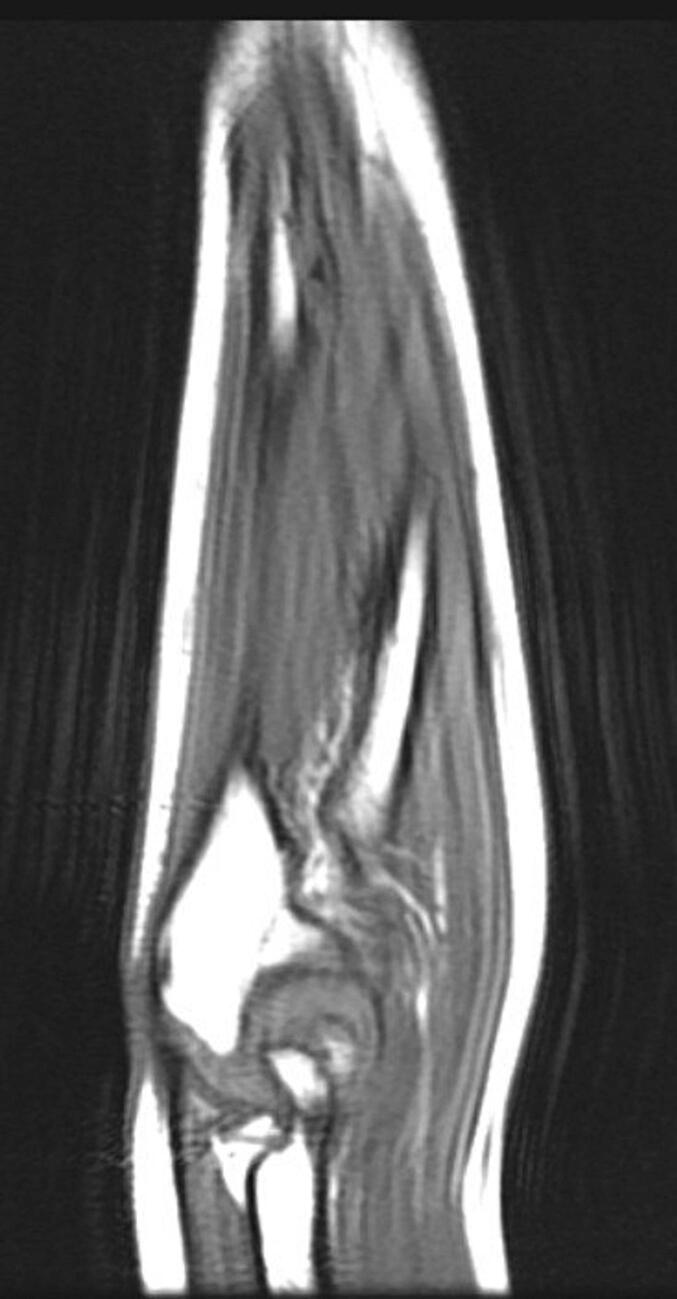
Preoperative MRI indicates the FCD at the distal ulna (frontal view).
